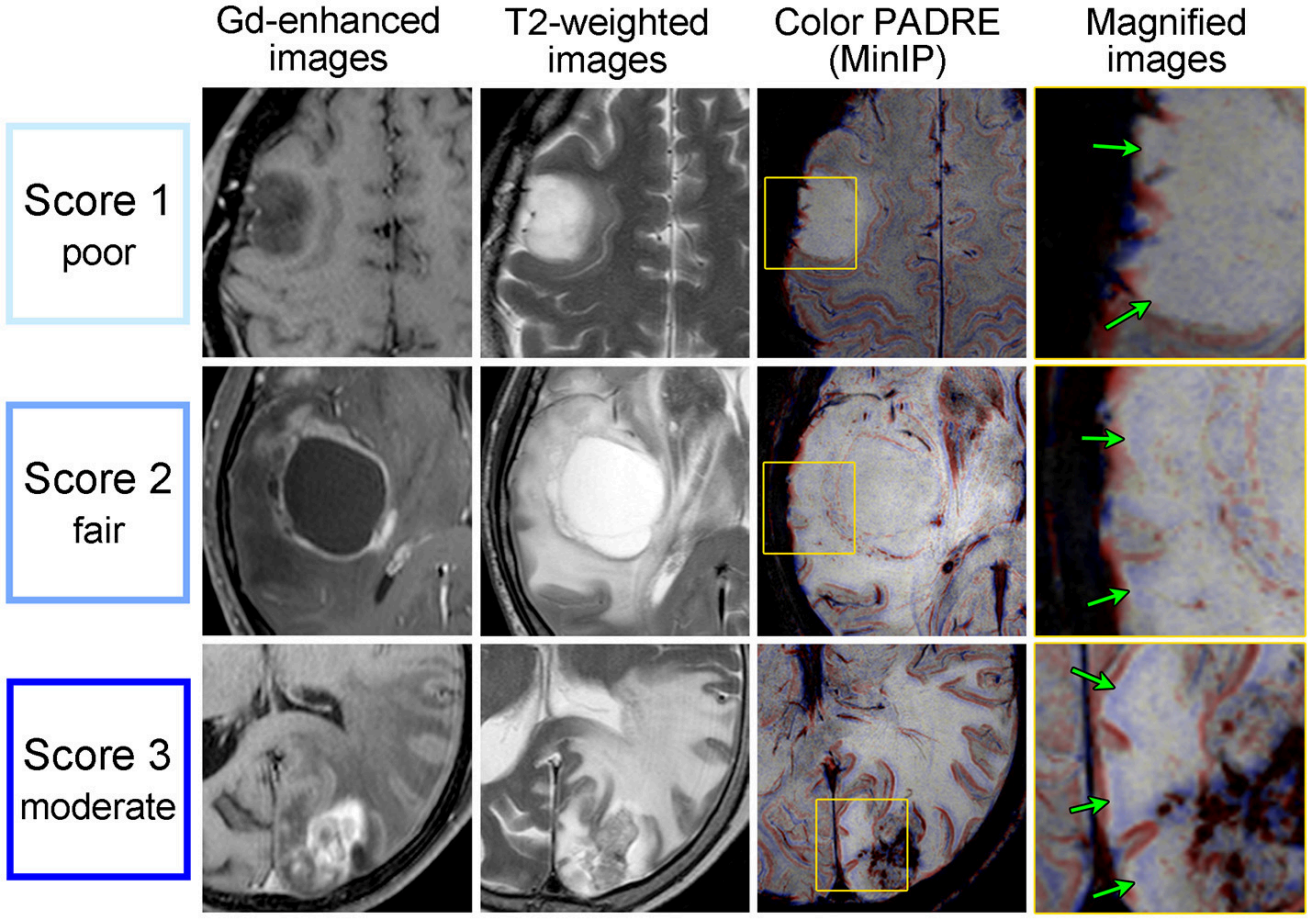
Gd-enhanced images (column 1), T2-weighted images (column 2) and Color PADRE (columns 3 and 4) of the representative cases with scores of 1–3 for SWM. The fourth column shows magnified images of the parts surrounded by yellow squares in the corresponding images in the third column. The first row shows score-1 images of a 43-year-old female with a DA; the second row shows score-2 images of a 67-year-old male with a GB; and the third row shows score-3 images of a 74-year-old female with a MT. No target area received a score of 4 regarding SWM. On Color PADRE, SWM is displayed as blue beneath the cortex. Green arrows in the fourth column indicate the SWM in non-Gd-enhancing T2-hyperintense regions.
